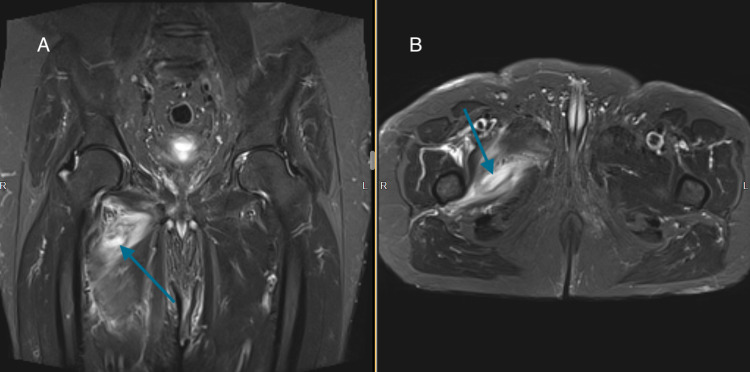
Gadolinium-enhanced T2 turbo inversion recovery magnitude (TIRM) MRI (A) coronal and (B) axial view with hip adductor muscle abscess on the right side (arrows).
